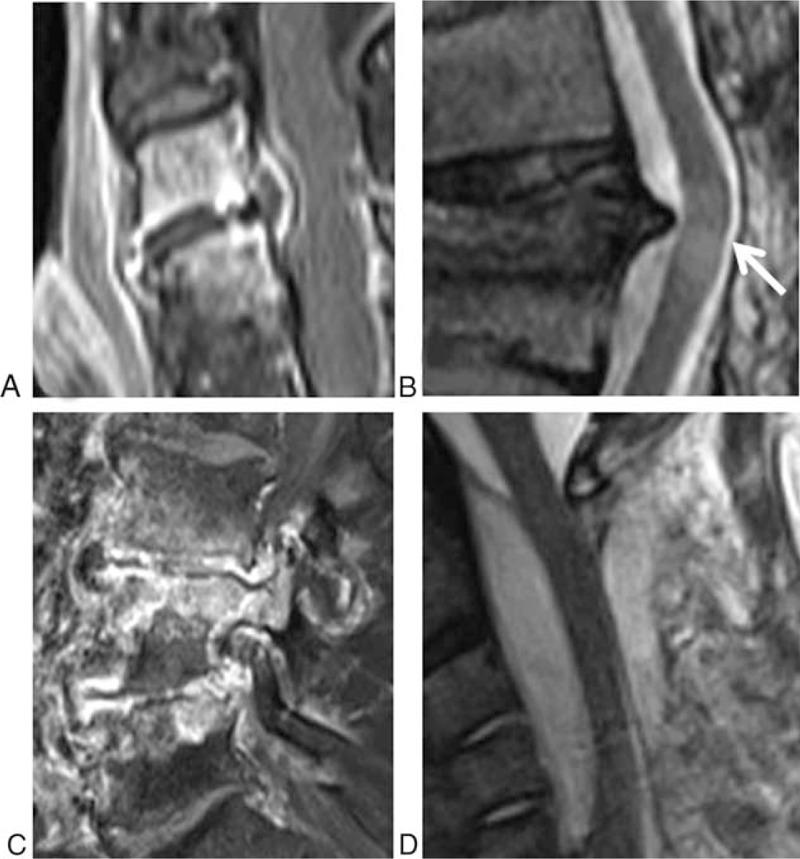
Examples of MRI abnormality: Epidural abscess in T1 weighted sagittal plane with fat saturation and gadolinium enhancement and compression of the dural sac (A), compressive myelopathy with spinal cord hypersignal (arrow) and anterior effacement of subarachnoid space in T2 weighted sagittal plane (B), edema and destruction of the posterior arch in T2 weighted sagittal plane with fat-saturation (C), voluminous epidural abscess with stenosis and anterior effacement of subarachnoid space in T2 weighted sagittal plane (D).
